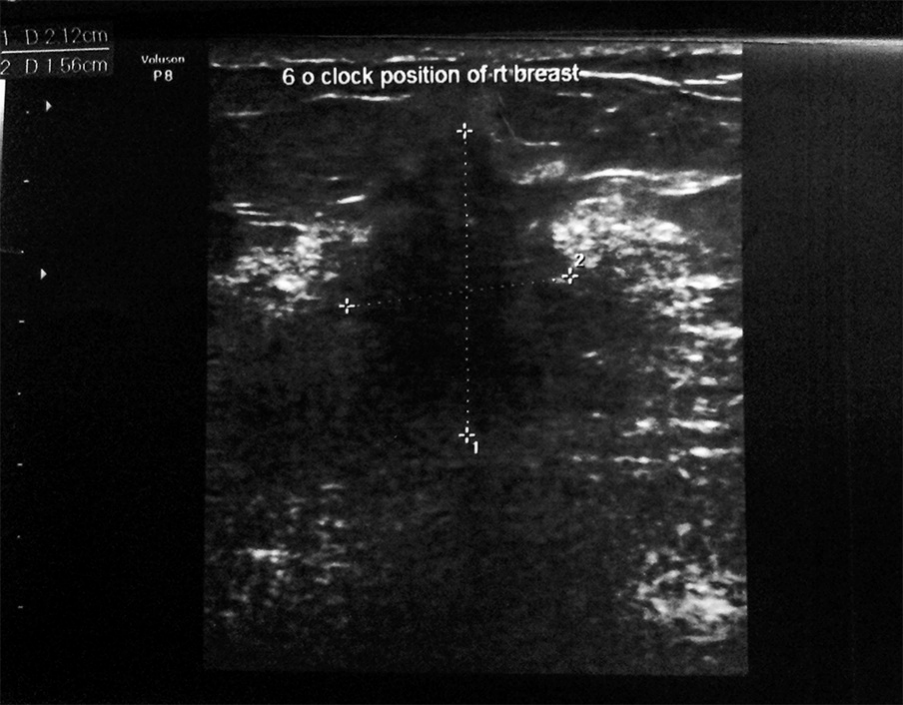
Right breast ultrasound. An ill-defined, hypoechoic, taller-than-wide lesion measuring 2.1 × 1.5 cm at the 6 o'clock position in the right breast.
